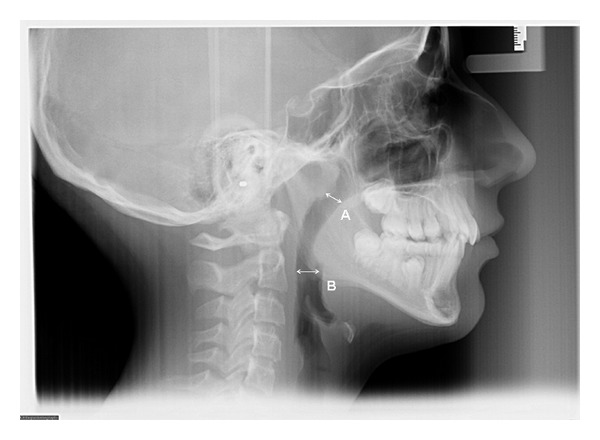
Measurements of the upper (A) and lower (B) pharyngeal airway widths were taken using tracing of the lateral cephalometric radiograph.
